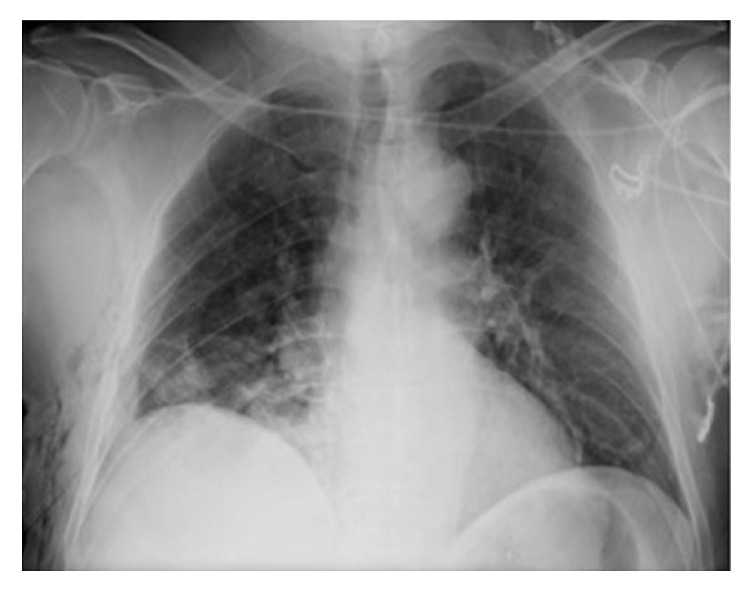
Chest radiography shows an enlarged cardiac silhouette.
